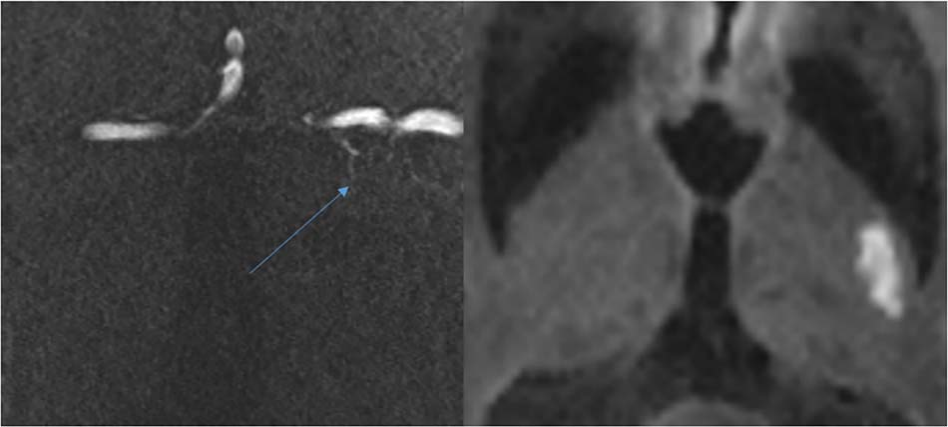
Small vessel occlusion (blue arrow) and possible associated diffusion-weighted imaging (DWI) hyperintensity on DWI sequence.
